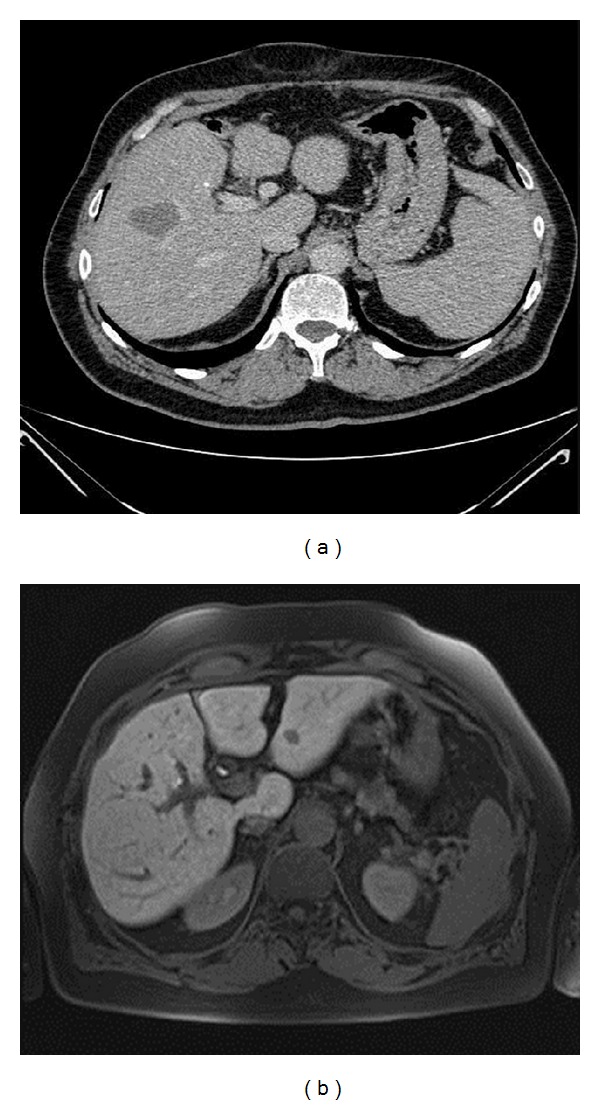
63-year-old man with an ablated HCC at segment 5–8. (a) Portal-phase CT scan at 1-month followup. The necrotic lesion is recognizable. (b) Hepatospecific phase MR image at 1-month followup. A new HCC is evident in the left lobe.
